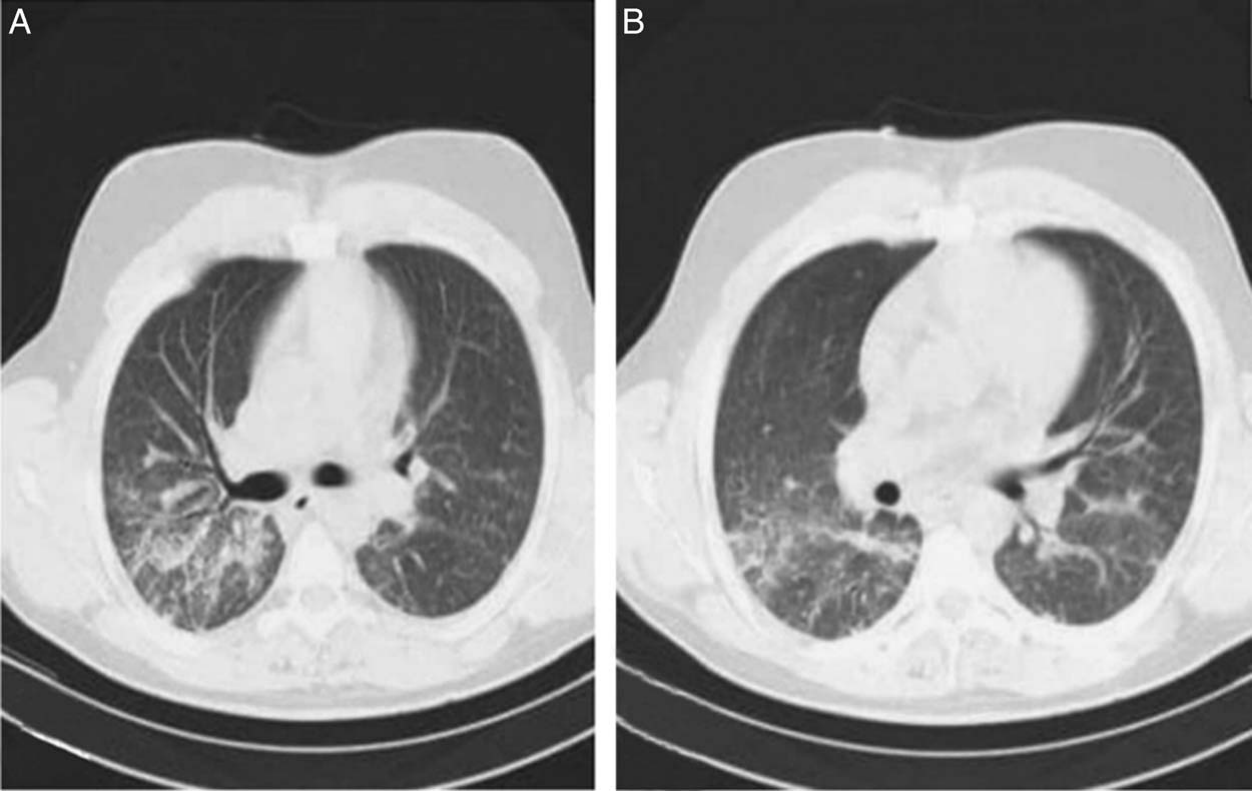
(A, B): Computed tomography scan showing diffuse pulmonary infiltrates most consistent with coronavirus disease 2019 and no presence for an aneurysm.
